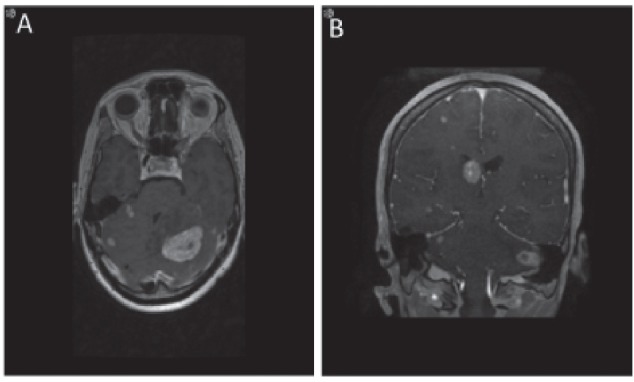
MRI brain demonstrating metastases to brain. Axial (a) and coronal (b) views of the brain demonstrating the presence of brain metastasis.
